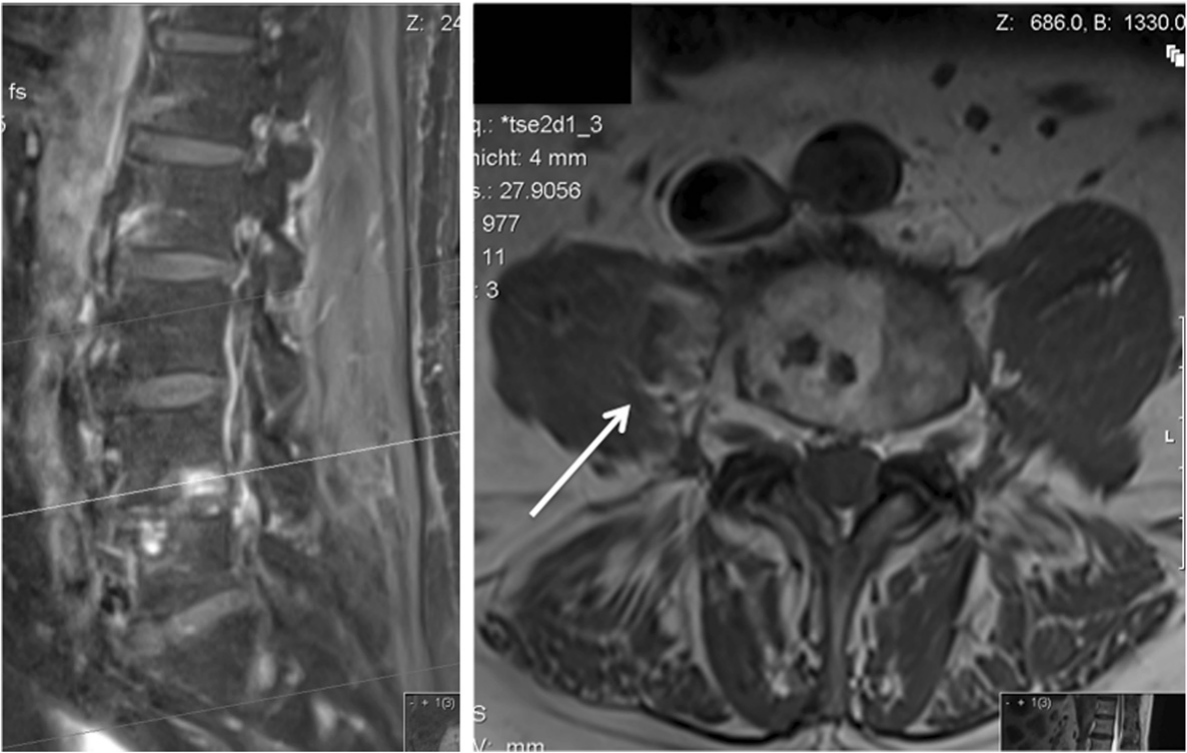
Sagittal (*left*) and axial (*right*) fat-suppressed T1-weighted MR images after IV infusion of 0.1 mmol/kg of gadoteric acid demonstrating an intramuscular abscess in the right psoas muscle at the L4/5 level (*white arrow*)
